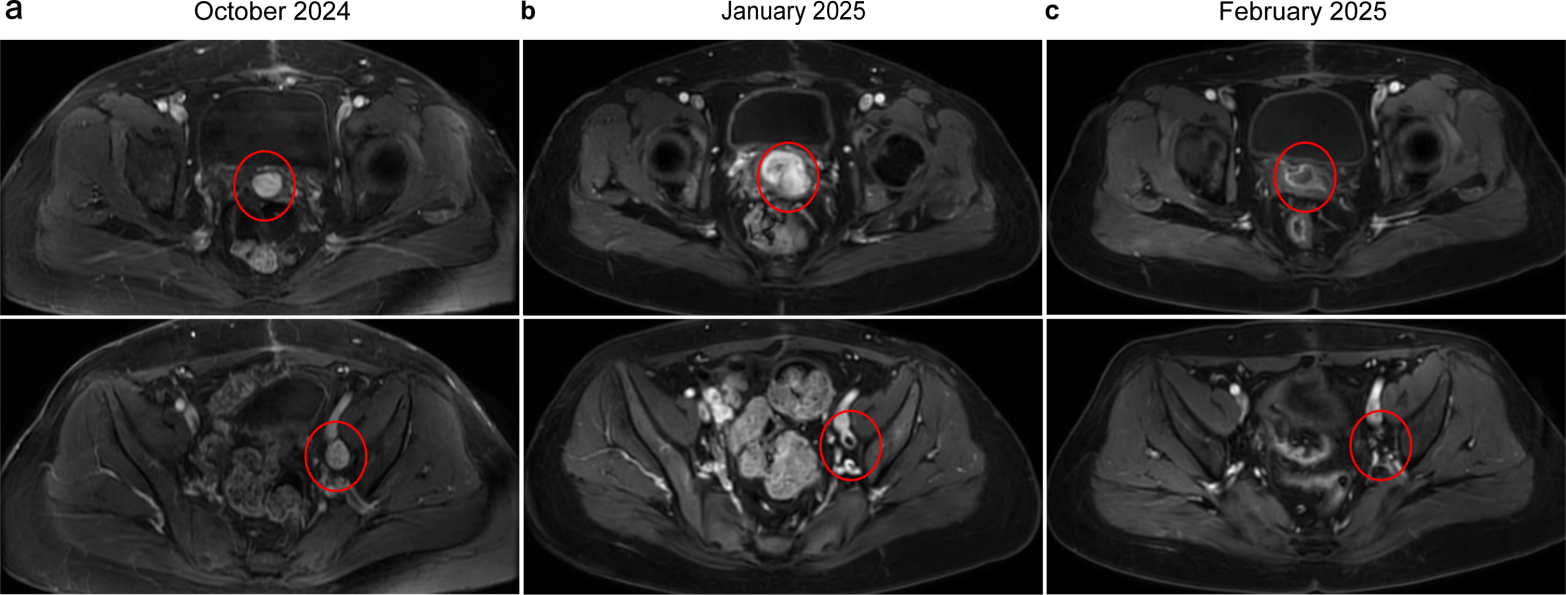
Baseline and follow-up contrast-enhanced MRI of the abdomen and pelvis. (a) Pre-chemotherapy images (October 25, 2024) reveal a new metastatic pelvic mass posterior to the bladder along with pelvic lymphadenopathy. (b) After chemotherapy (January 13, 2025), the pelvic lymph node metastases show shrinkage; however, the metastatic lesion behind the bladder exhibits 90% enlargement. (c) Following the initiation of Iruplinalkib treatment, both the pelvic metastatic lesion and lymph node metastases demonstrate a significant radiographic response (February 24, 2025).
